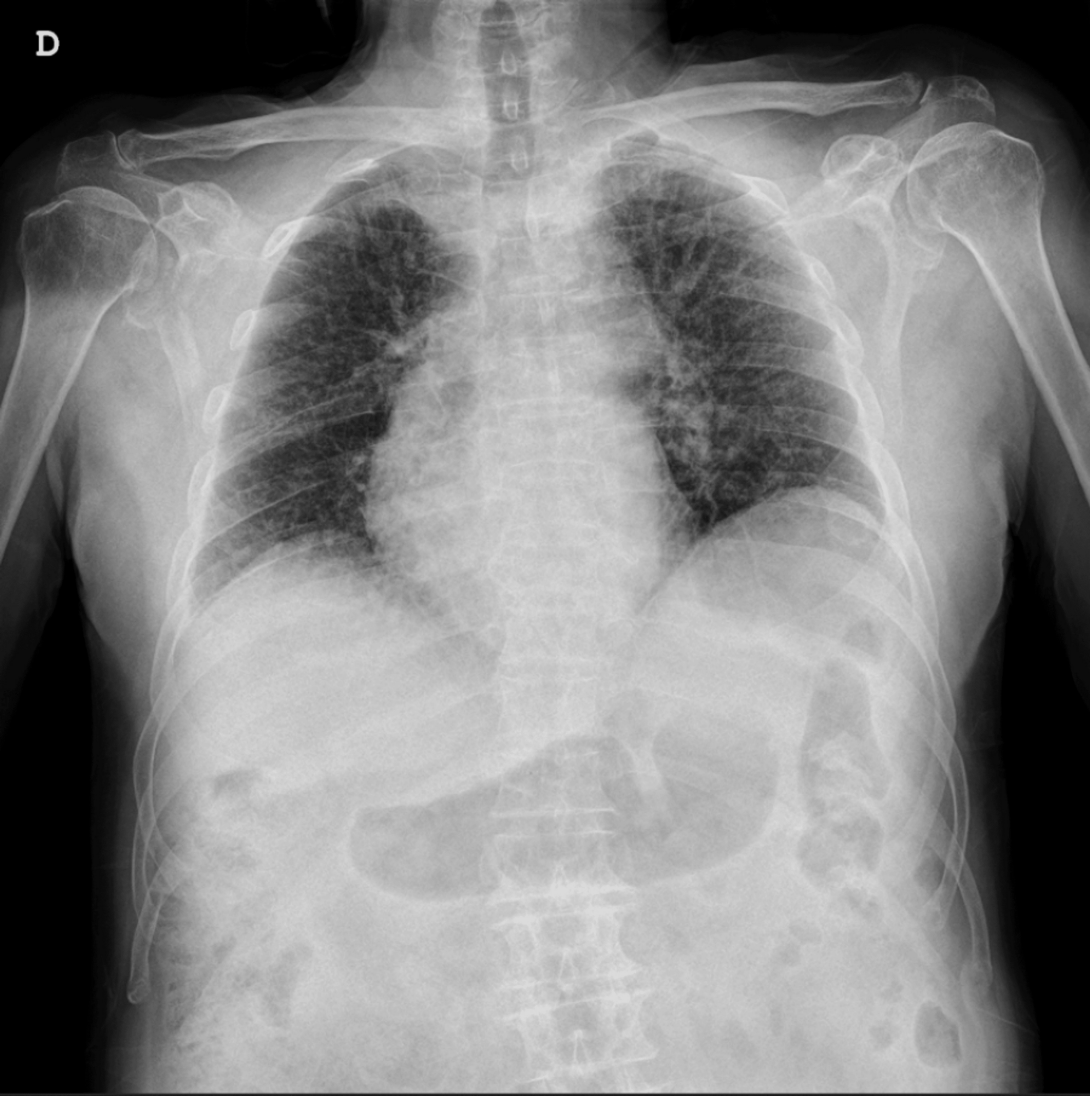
Chest X-ray after diuretic therapy
